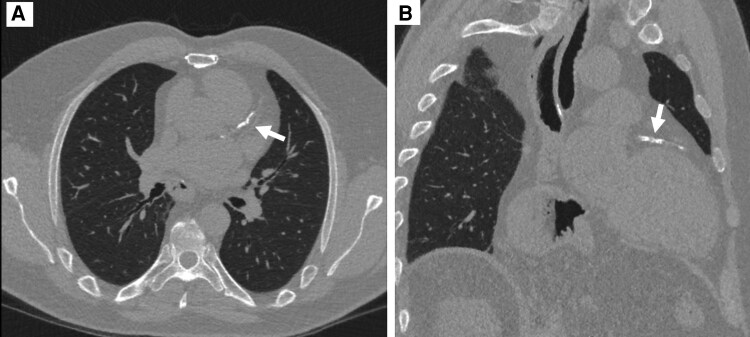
Thoracic computed tomography (CT) in a 52-year-old male with colon adenocarcinoma. The CT scan performed for tumour staging revealed extensive calcification of the left anterior descending coronary artery.
